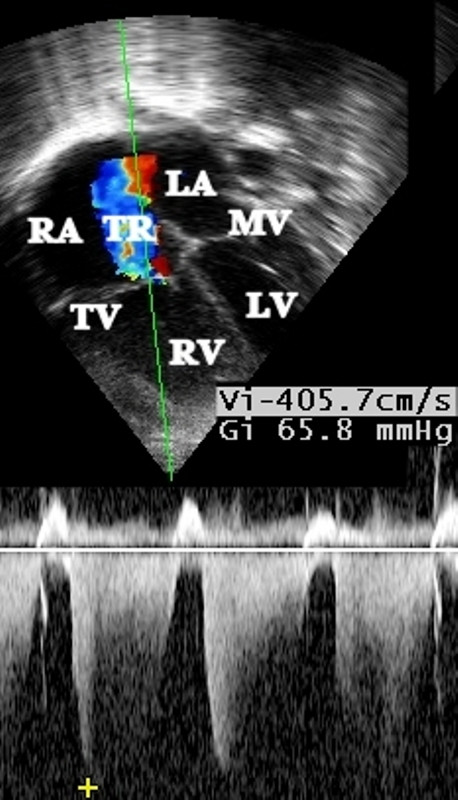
Echocardiogram Doppler gradient in a four-chamber view demonstrating a gradient of 65 mmHg from right ventricle to right atrium as measured by the tricuspid regurgitation jet (LA: left atrium, MV: mitral valve, LV: Left ventricle, RA: right atrium, TV: tricuspid valve, RV: right ventricle, TR: tricuspid regurgitation jet).
